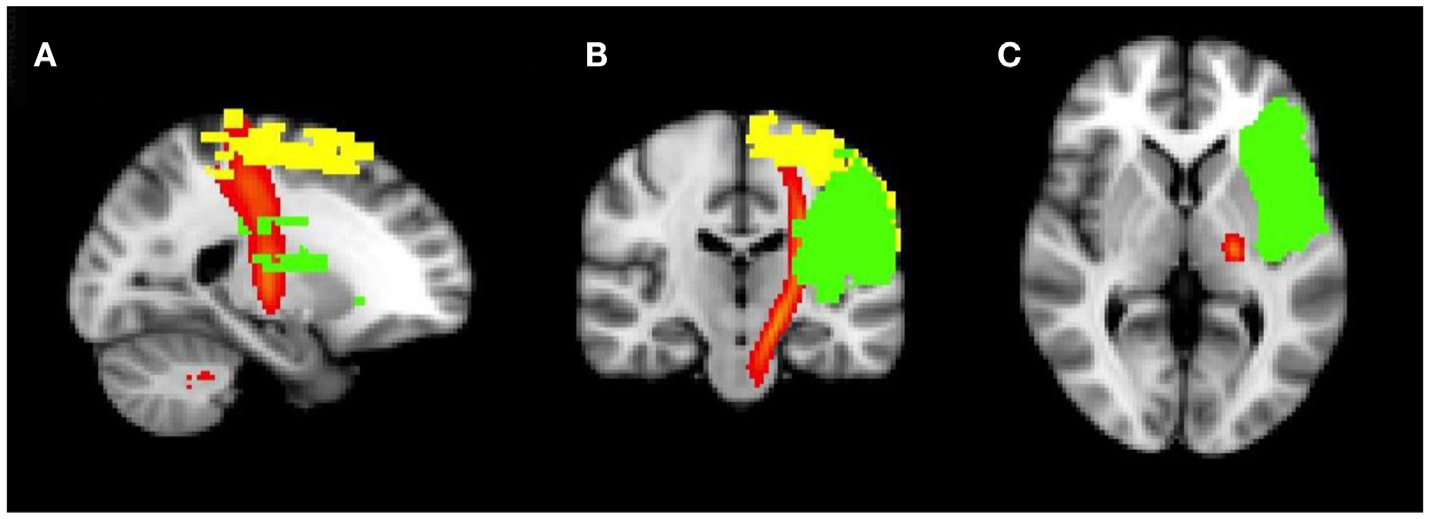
Structural MRI (FLAIR sequence). Overlay of normalized lesion maps of patients 2 and 3 in the standard brain. Yellow, Patient 2 (Base: M−/L+); green, Patient 3 (Base: M+/L−); red, corticospinal tract. (A) sagittal view; (B) coronal view; (C) axial, subcortical view.
